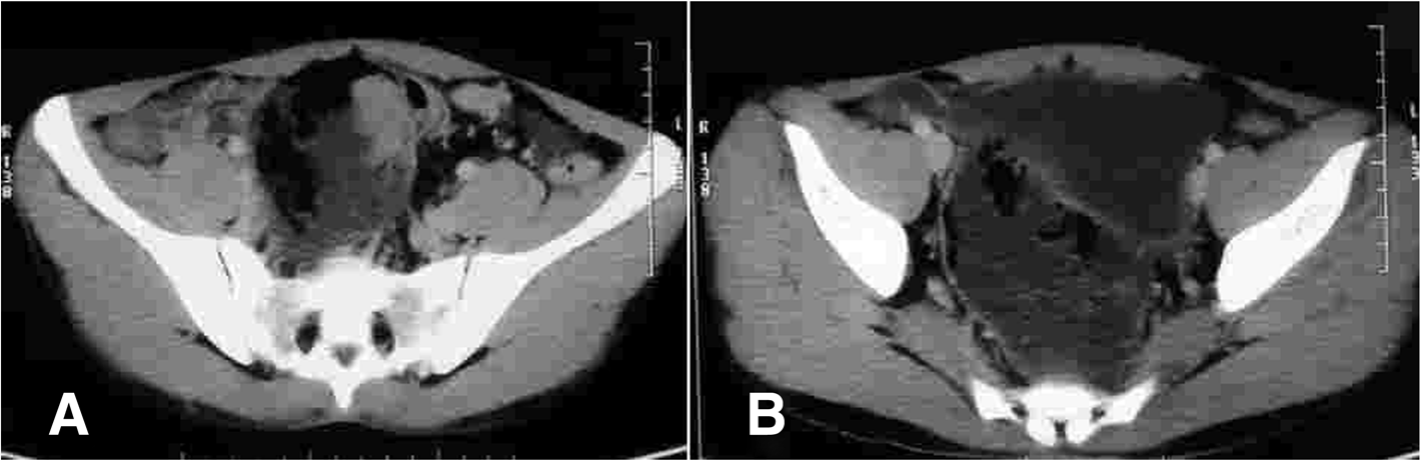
Computed tomographic images showing a) tumor at rectosigmaoid junction b) lesion in upper rectum
